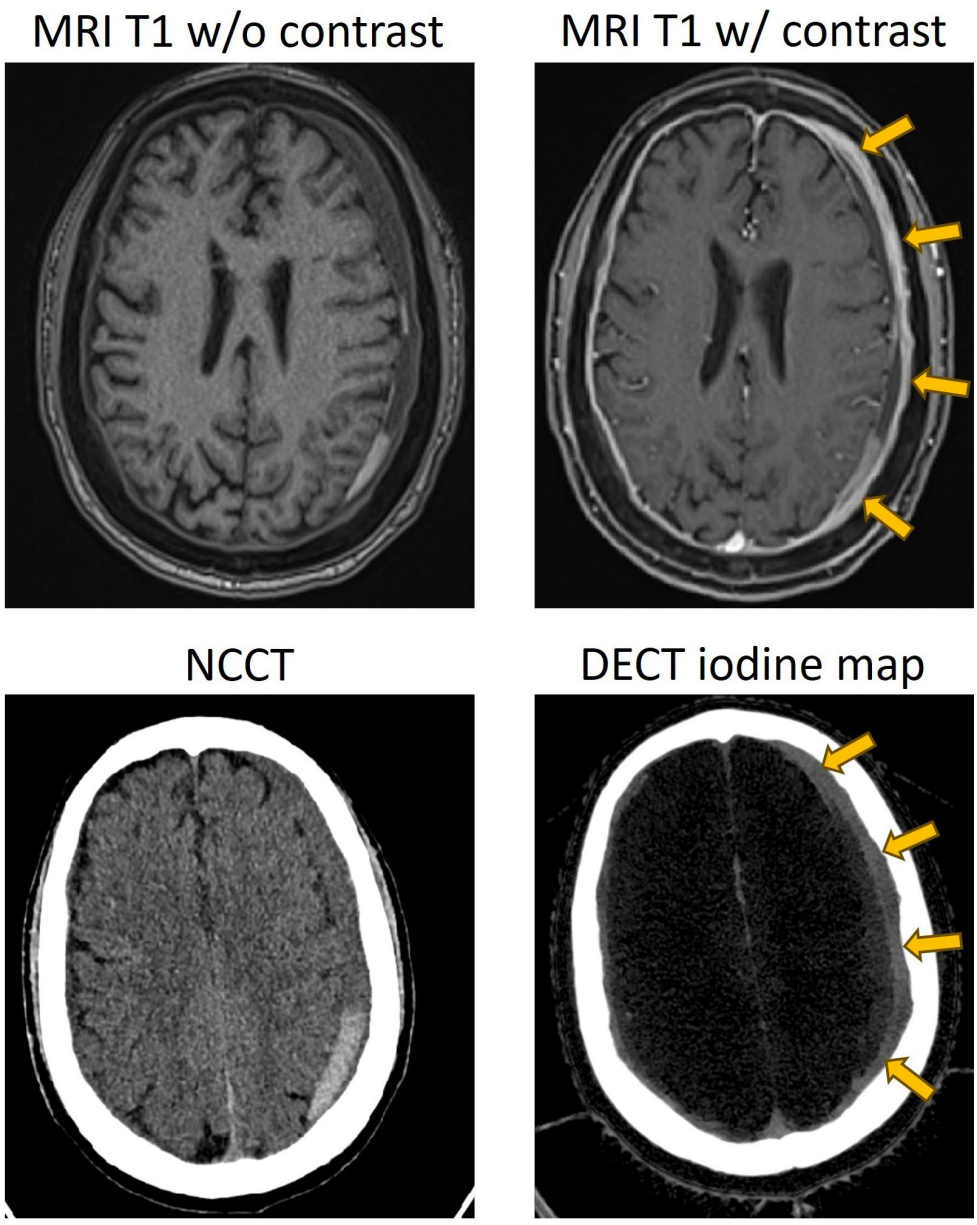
Representative case of a separated type of cSDH, with contrasted MR and dual-energy CT showing enhancement of the external membrane of a subdural hematoma (yellow arrows). NCCT, non-contrast CT; DECT, dual-energy CT.
